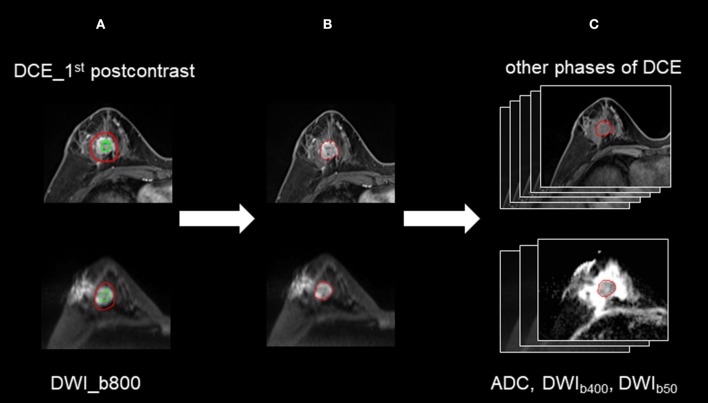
The process for 3D segmentation. The segmentation process comprised three steps: (A) The foreground and background seed points were manually drawn inside and outside the tumor on the DWI images with a *b*-value of 800 s/mm^2^ (DWI_b800_) and the first postcontrast of the DCE images, respectively. (B) The whole-tumor segmentations on DWI_b800_ and the first postcontrast of the DCE images were executed with a semiautomatic algorithm. (C) For DWI, 3D segmented contouring that was created on DWI_b800_ was propagated to the DWI images with other *b*-values. For DCE, 3D segmented contouring that was created on the first postcontrast images was propagated to the precontrast and four postcontrast phases of the DCE images.
